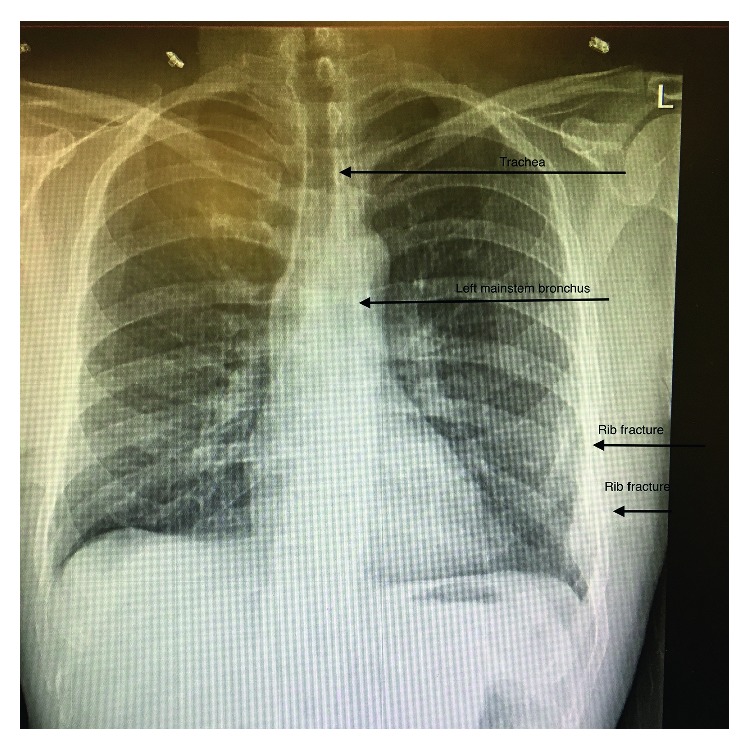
Chest radiograph from day 28 demonstrating the normal airway anatomy as well as the two left rib fractures.
